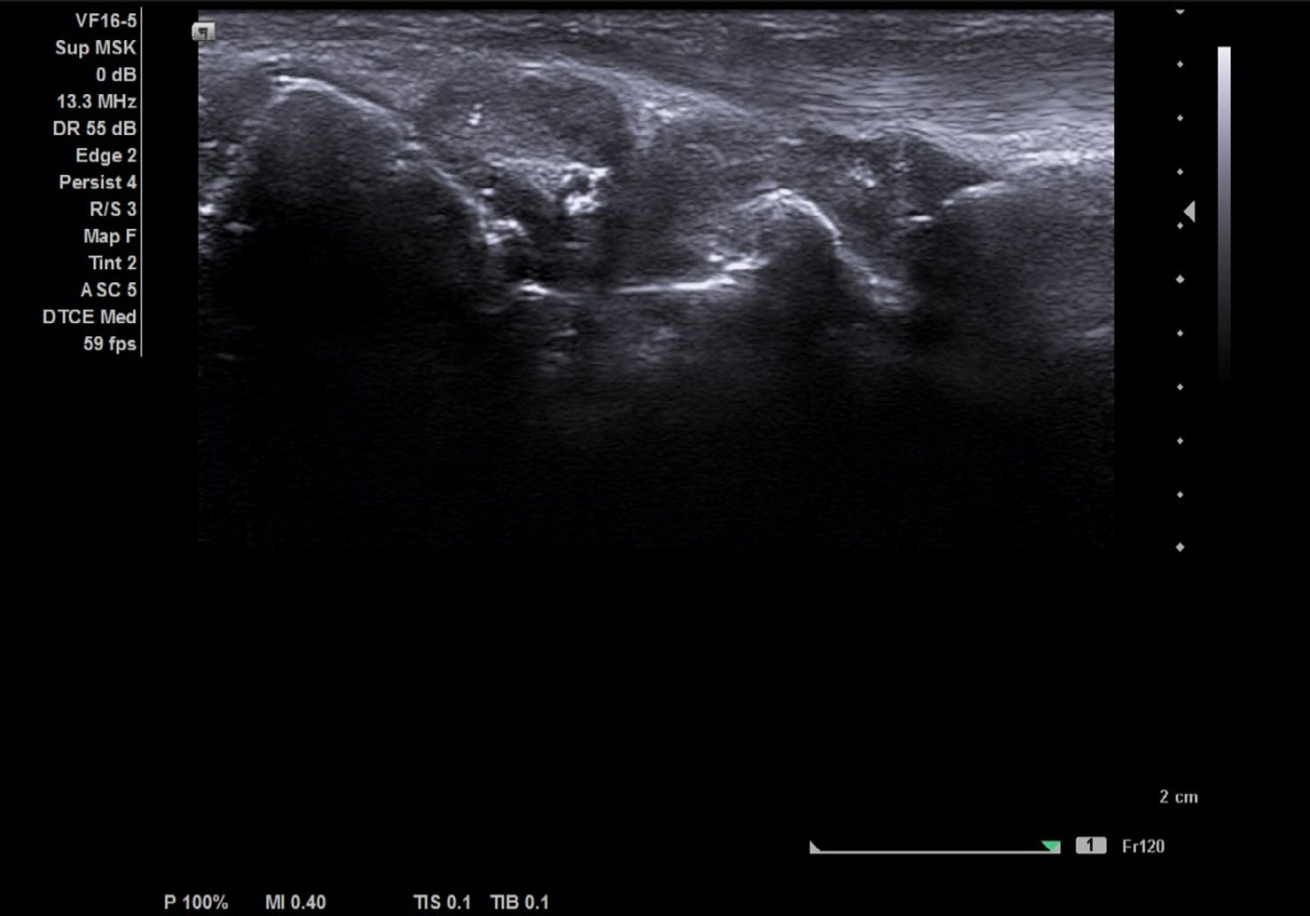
Ultrasound picture of radiocarpal joint on gray scale. Longitudinal ultrasound image of the radiocarpal joint showing the distal radius (left), lunate (center), and capitate (right) as hyperechoic cortical lines. A marked anechoic synovial effusion with synovial hypertrophy (grade III synovitis) is visible over the joint surfaces.
